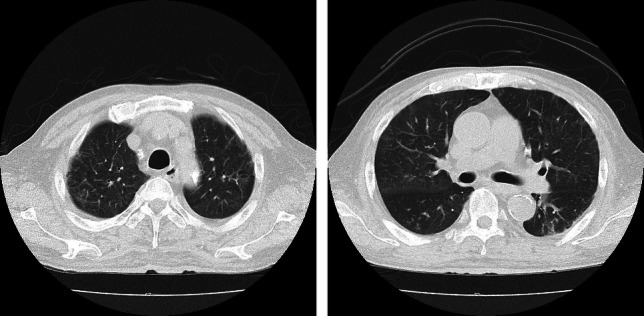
A computed tomography scan on day 10 of hospitalization shows resolution of ground-glass opacities
